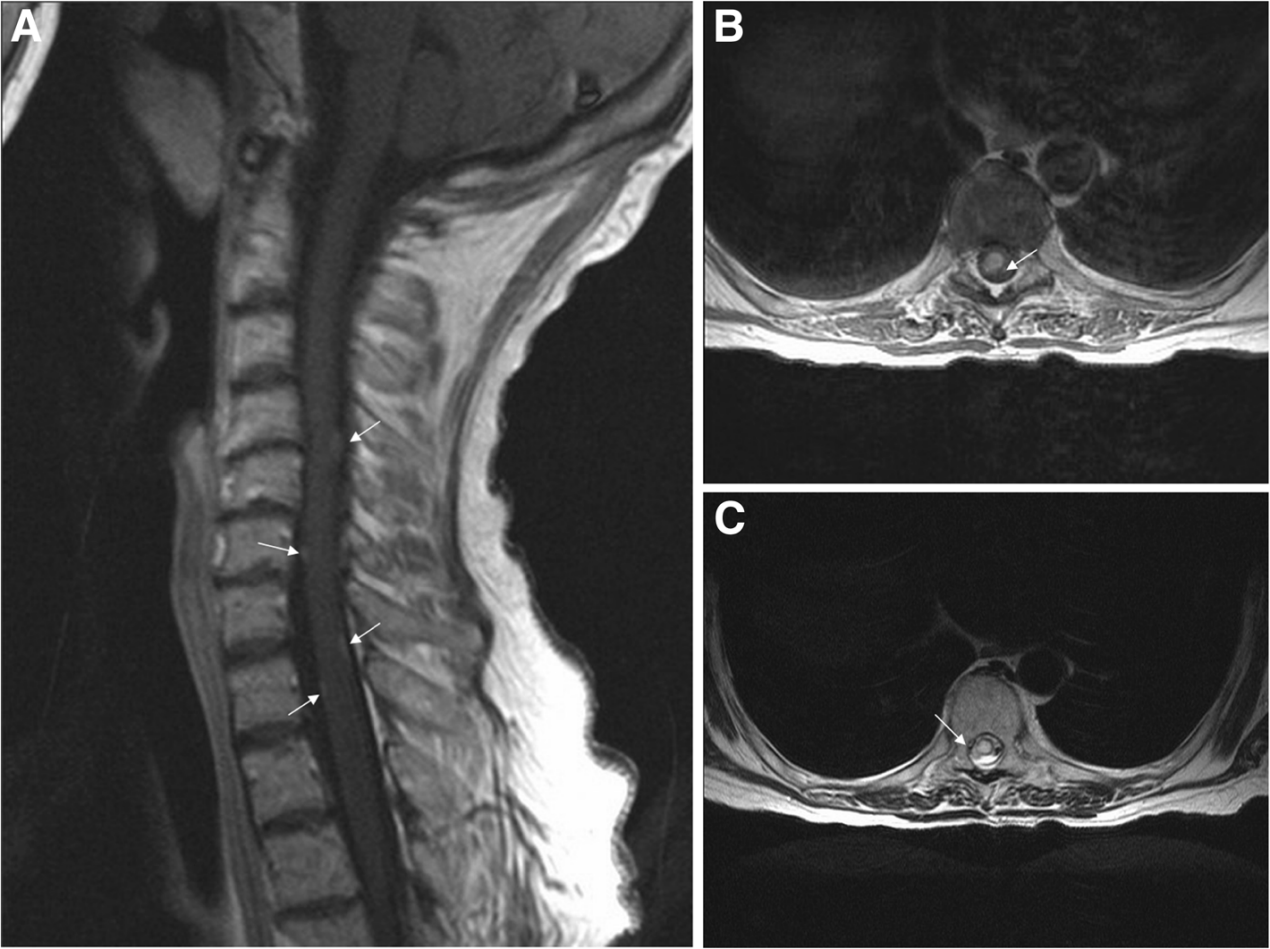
Radiological evaluation of the spinal cord. MRI of the cervical spine demonstrated an enhancing cervical leptomeningeal metastases (arrows) at the level C3 to C7. (A, T1-weighted image). Additionally, at the T8 to T10 level, MRI also revealed intramedullary metastases with extensive contrast enhancement, central necrosis and vasogenic edema (arrows) (B, T1-weighted image) and C (T2-weighted image).
